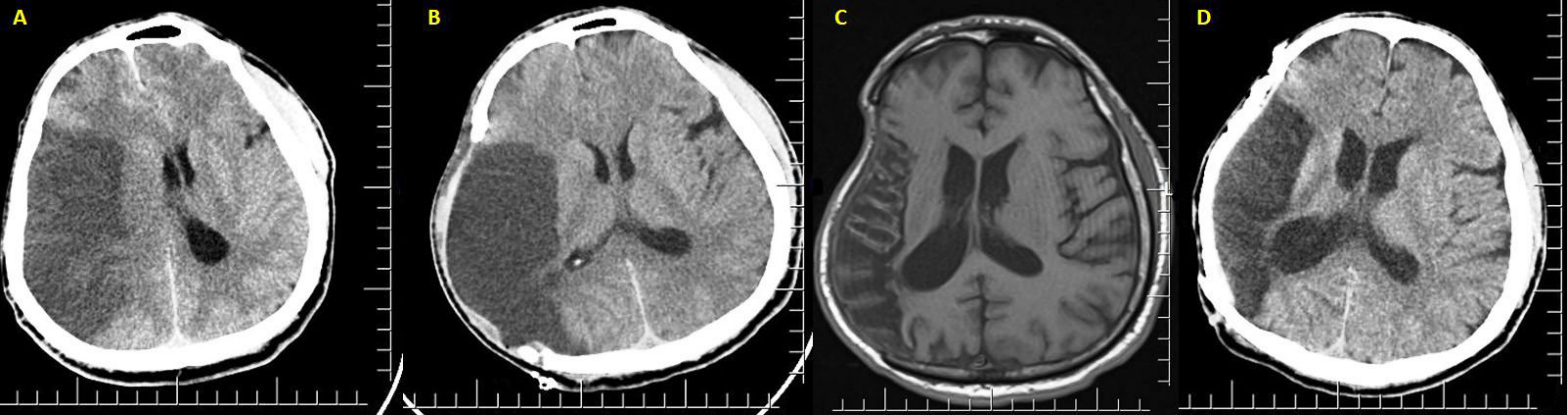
A 45-year-old patient underwent DHC due to right MCA infarction. Upon arrival at the hospital, his GCS was 14 and he had right MCA infarction, shift and edema were observed on CT (A). Herniation improved after DHC (B). Encephalomalastic area was observed on MRI 6 months later (C), and cranioplasty was performed (D). *DHC: Decompressive hemicraniectomy, MCA: Middle cerebral artery, GCS: Glascow coma scale, CT: Computed tomography, MRI: Magnetic resonance imagine.
